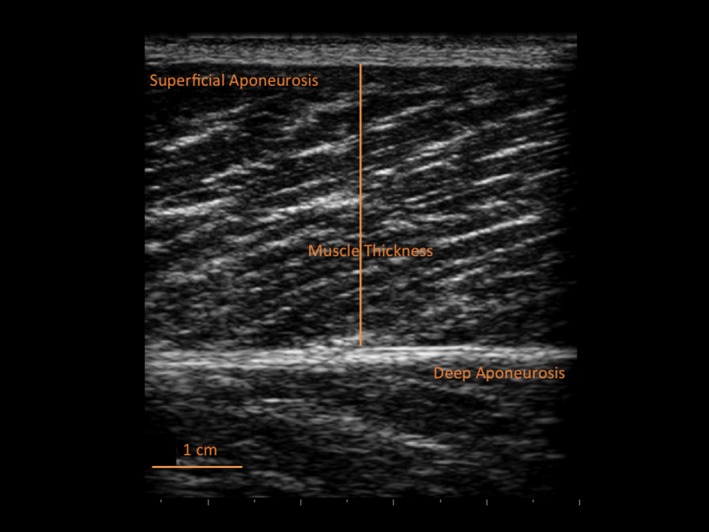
Ultrasound image of the *vastus lateralis* muscle (at 50% of femur length) from a representative subject with the muscle thickness measurement highlighted (solid line between aponeuroses)
